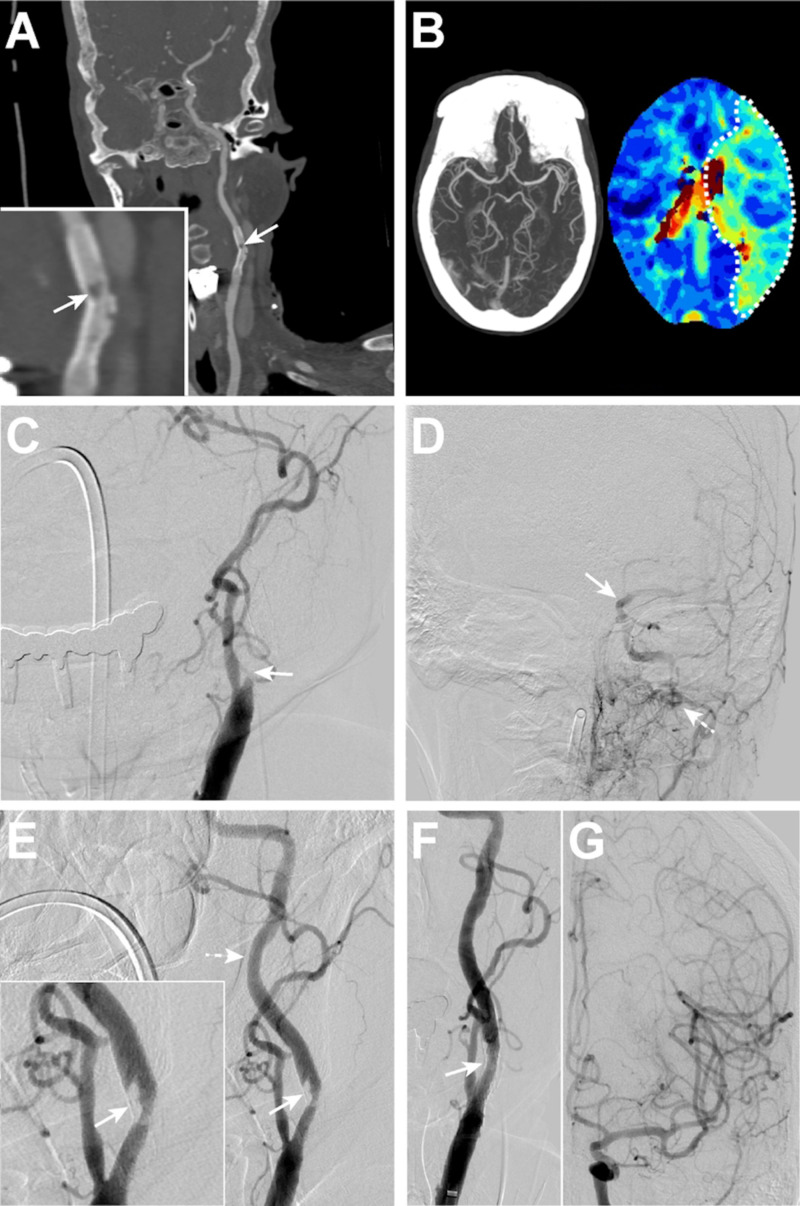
Patient 1 with left CSO seven days post stent placement (A) Coronal maximum-intensity-projection images following a CTA demonstrates a thrombus within the left internal carotid artery stent (arrow). Inset shows magnified region of stent with thrombus (arrow). (B) Left: Maximum intensity projection of the Circle of Willis CTA demonstrates no intracranial large vessel tandem occlusions. Right: CT perfusion imaging shows a perfusion deficit (Tmax >6 seconds) in the left middle cerebral artery territory (dashed outline) secondary to the carotid stent thrombus. (C-G) Left common carotid artery DSA images. There is occlusion of the left carotid stent (c, arrow) with no antegrade filling of the cervical left ICA (c) and poor filling of the intracranial left ICA (d, arrow), largely via left external carotid artery collaterals (d, dashed arrow). (E) CSO treatment by aspiration thrombectomy resulted in antegrade filling of the left ICA stent with residual non-occlusive thrombus within the stent (arrow; inset, arrow) and improved filling of the more distal cervical ICA (dashed arrow). (F) Subsequent angioplasty resulted in minimal residual thrombus within the stent (arrow). (G) Robust antegrade filling of the left anterior circulation was present after CSO treatment. CTA, computed tomography angiography; ICA, internal carotid artery; DSA, digital subtraction angiography; CSO, carotid stent occlusion
